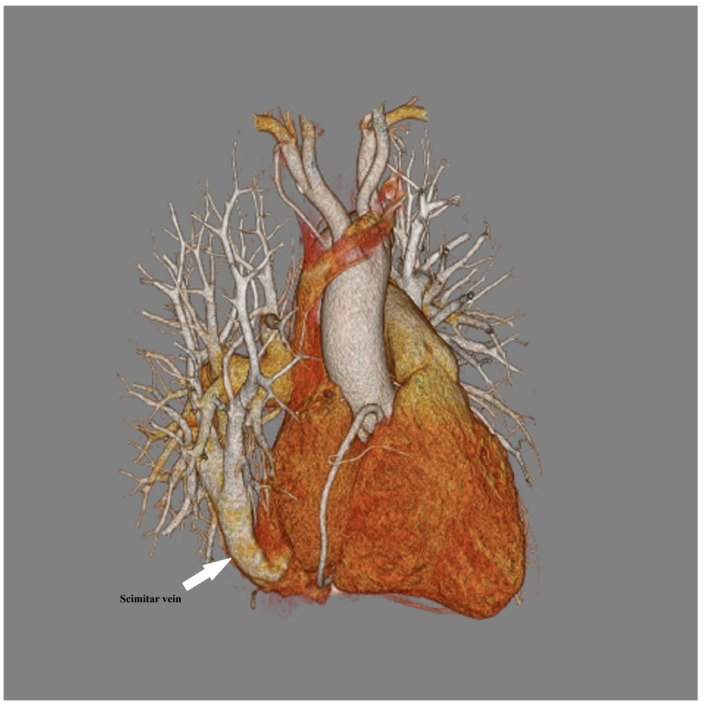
Three-dimensional computed tomography (CT) reconstruction demonstrating anomalous right pulmonary venous drainage in a patient with Scimitar syndrome. The scimitar vein (arrow) is visualized coursing inferiorly along the right cardiac border.
